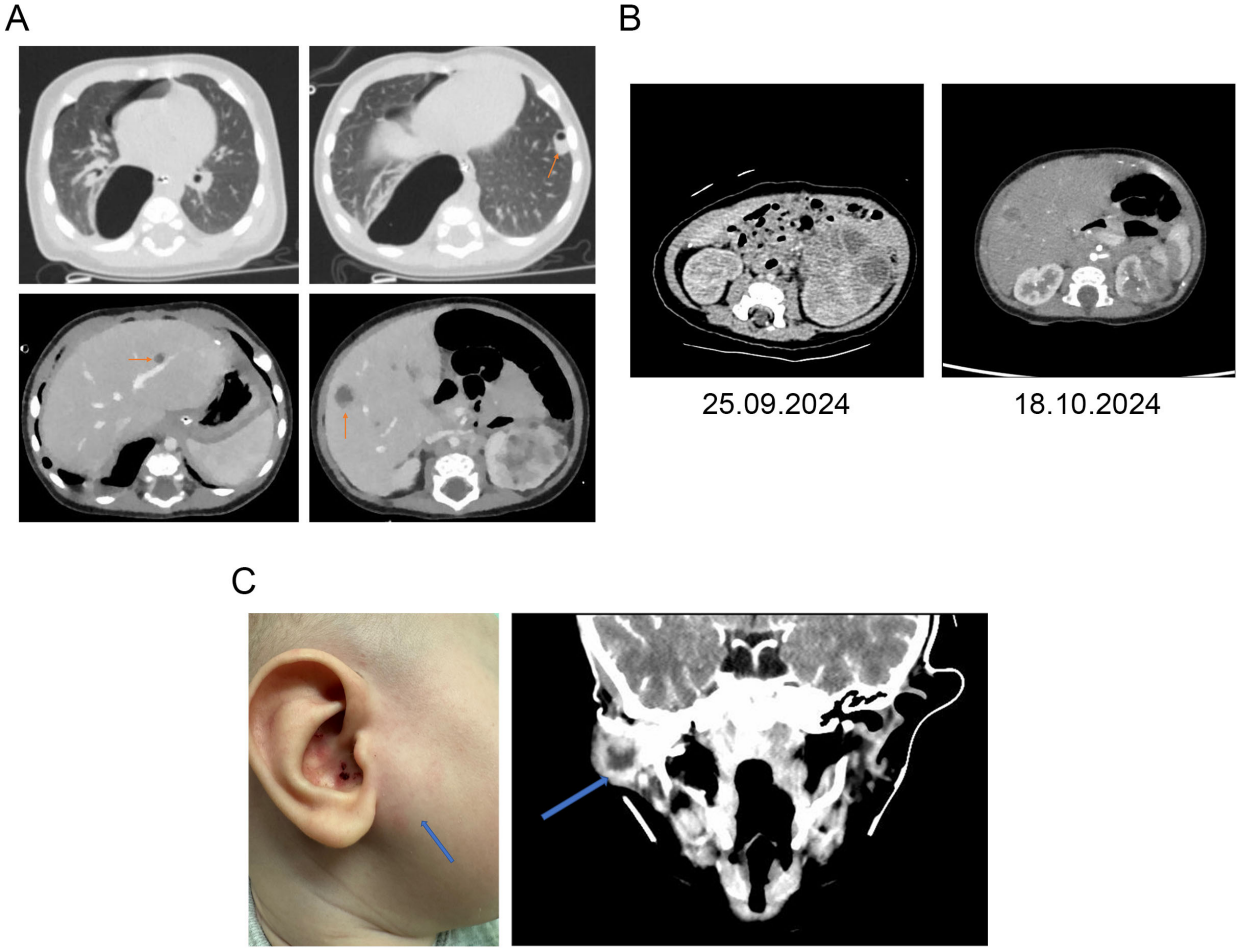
Radiological findings of patient. (A) CT scan of thoracic and abdominal organs. (B) CT of kidneys over time. (C) External view of cervical lymph node abscess and cervical CT scan.
